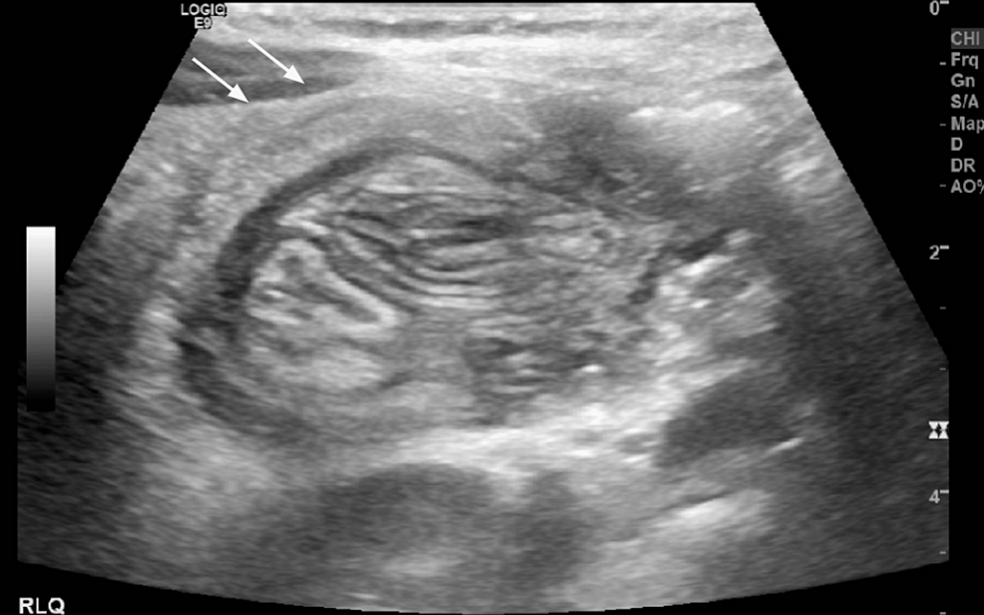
Abdominal ultrasound image one hour after the reduction The image shows recurrent ileocolic intussusception to the level of the hepatic flexure (white arrows).
